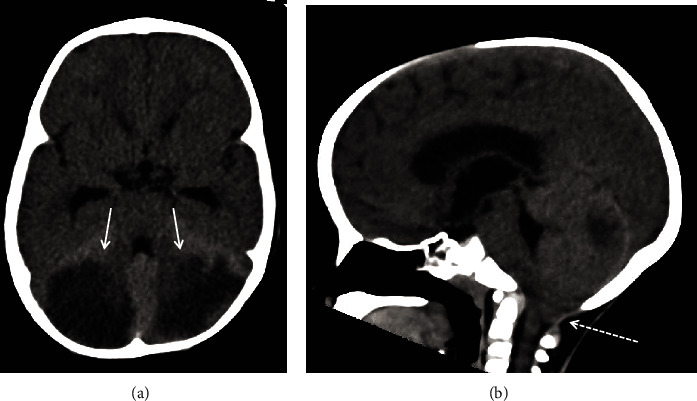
Axial (a) and midsagittal (b) noncontrast head CT at presentation shows bilateral cerebellar hemisphere hypoattenuation (low density) with swelling (arrows). There is inferior herniation of the cerebellar tonsils with crowding at the foramen magnum (dashed arrow).
